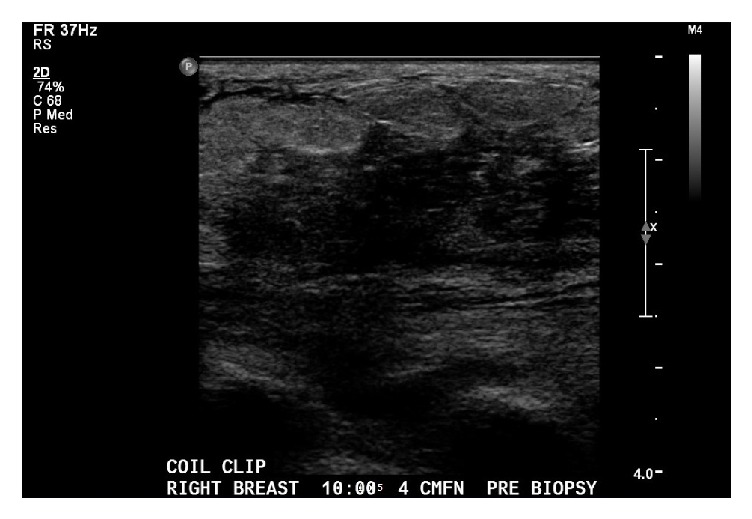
Ultrasound of ill-defined breast lesion showing hypoechoic mass with irregular borders. Ultrasound interpreted as BI-RADS 4, suspicious abnormality with differential diagnoses of phlegmon, malignancy, and calciphylaxis. Surgical consultation recommended.
